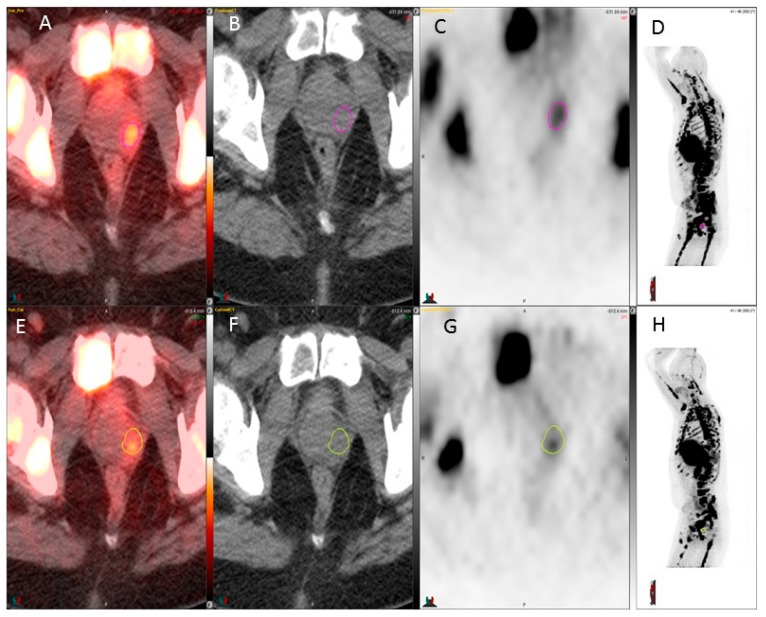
^18^F-FLT used for detection of prostate foci before and after chemotherapy. A weak response is seen, while the SUVmax value decreased from 3.6 to 2.3 in the left prostate lobe (circles in C and G). Upper row, baseline: from the left fusion image (A), computerized tomography (CT) image (B), fluorine-18 fluorothymidine with positron emission tomography (^18^F-FLT PET) image (C); and maximum intensity projection (MIP) image (D); lower row, after 4 weeks of therapy: from the left fusion image (E), CT image (F), ^18^F-FLT PET image (G), and MIP image (H). Color bar range from 0 to 5.
